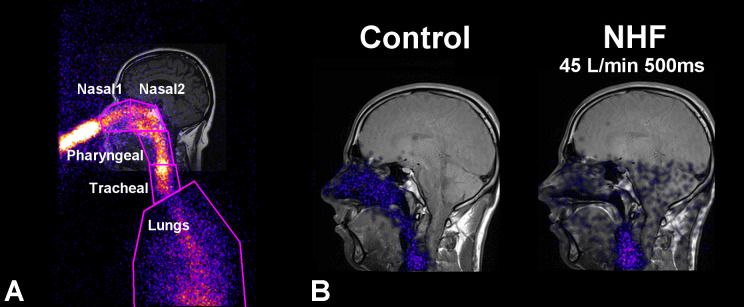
Lateral gamma camera image of nasal ^81m^Kr gas inhalation overlaid on the coronal MRI image of a volunteer during breath holding. *A*: definition of anterior (Nasal1), posterior (Nasal2), pharyngeal, tracheal, and lung ROIs. *B*: visualization of ^81m^Kr gas distribution 500 ms after the application of NHF at a rate of 45 l/min (*right*) compared with the control (*left*) shows fast clearance of the tracer gas in the upper airways. The control measurement without cannula flow shows stable ^81m^Kr gas concentration.
